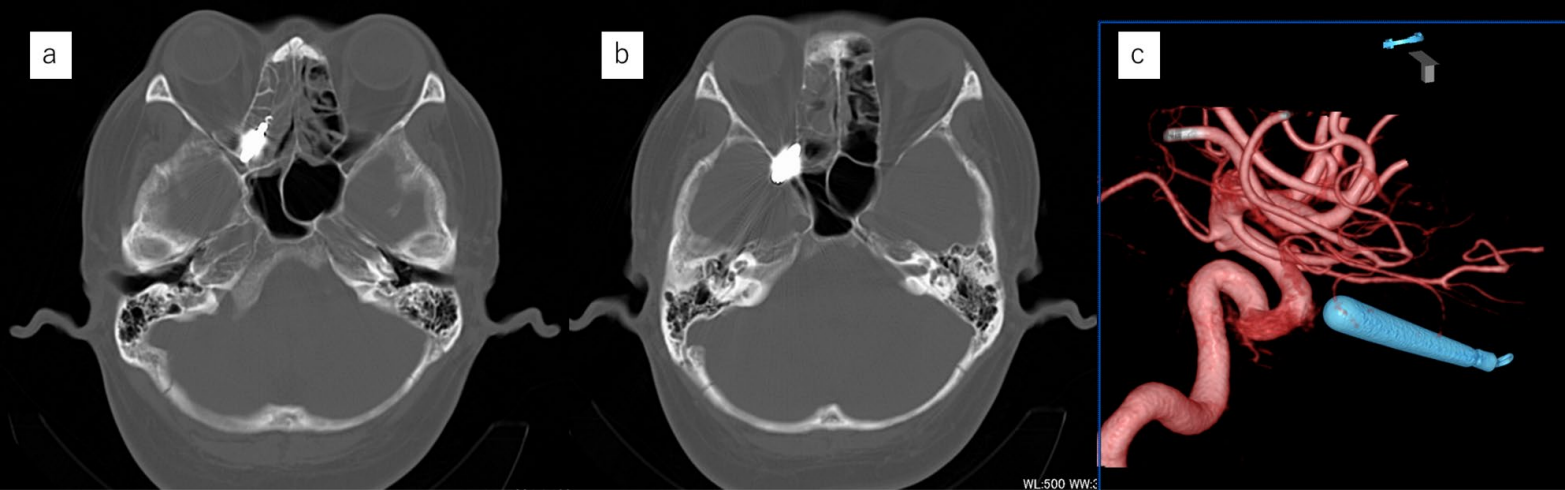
Computed tomography (CT) images. (a and b) Axial CT images showing a foreign body located in the paranasal sinus and penetrating the orbit and middle cranial fossa. (c) Cerebral angiography showing the foreign body located away from the internal carotid artery.
